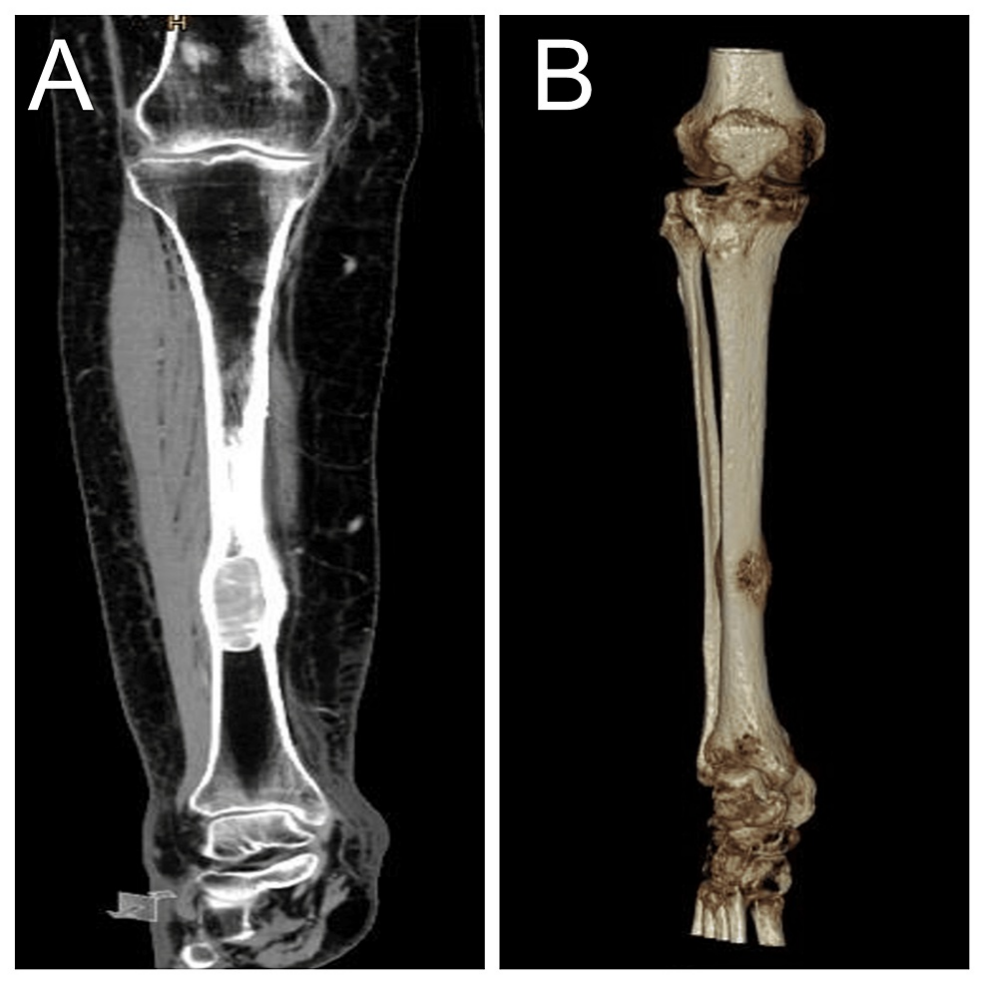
Tibia/fibula computed tomography (A) bone window (B) three-dimensional view showing a well-defined, slightly expansile, oval-shaped, intramedullary osteolytic lesion in the distal third of the diaphysis of the right tibia.
